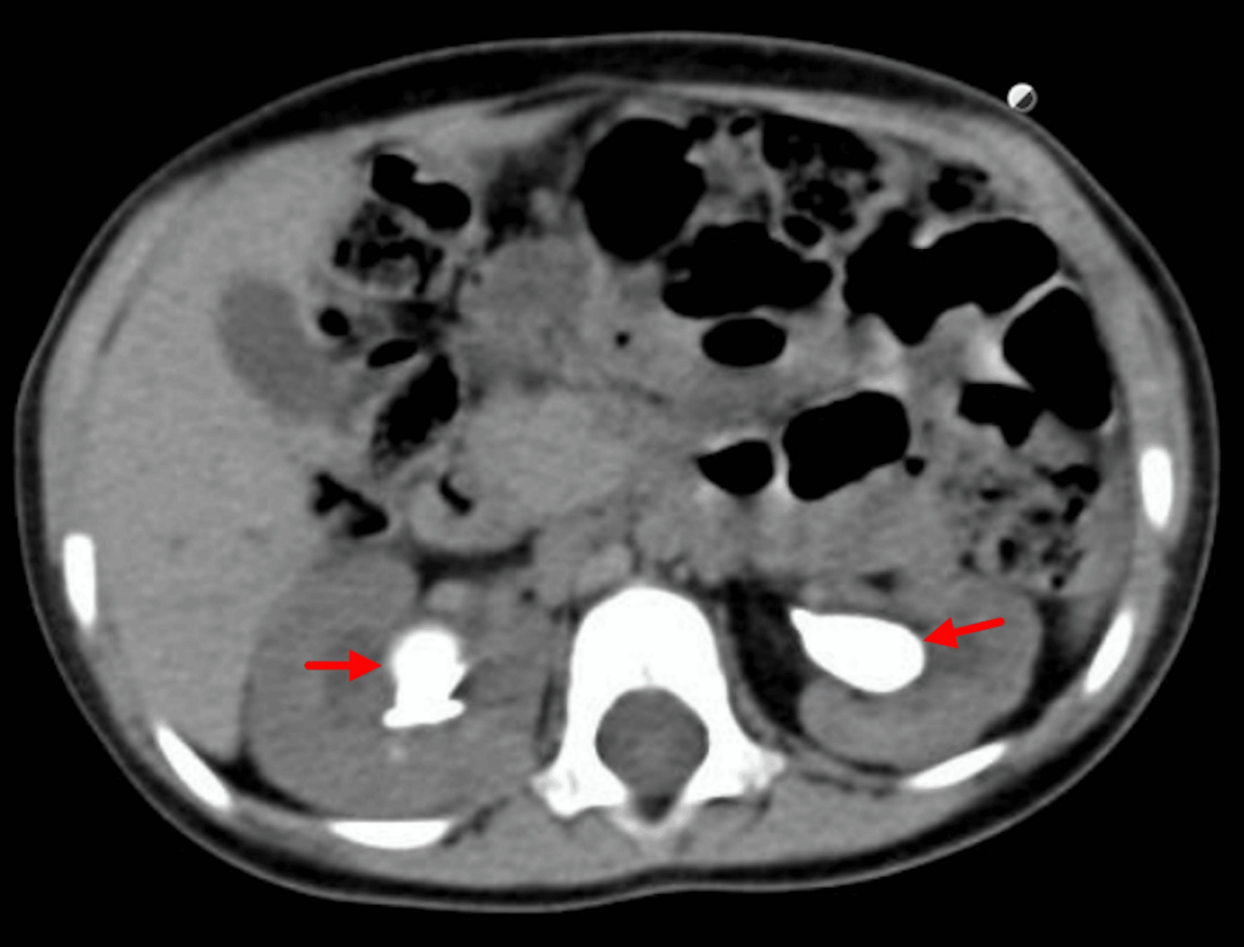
Axial view of non-contrast-enhanced CT of the abdomen and pelvis Bilateral staghorn calculi are indicated by the red arrows.
